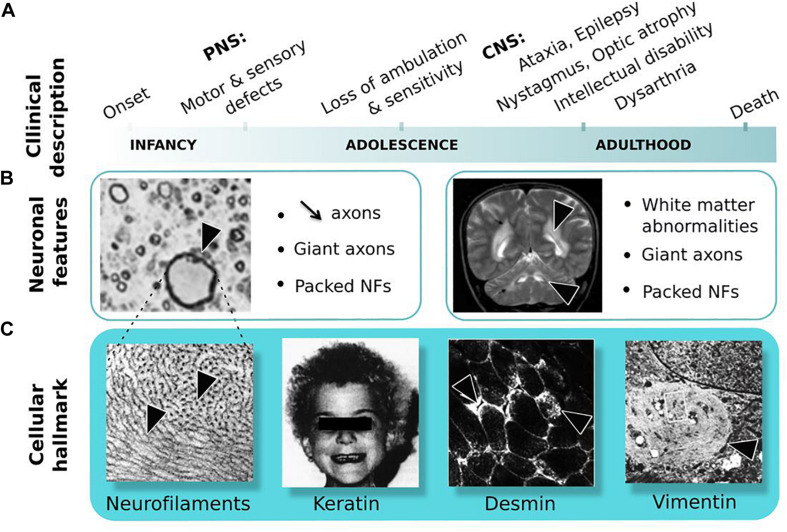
Giant Axonal Neuropathy. (A) Symptoms are shown in order of occurrence: from onset in childhood in the peripheral nervous system (PNS) to adulthood in the central nervous system (CNS). (B) Neuronal features: (left) nerve cross-section in the PNS shows reduced axonal content and giant axon; (right) magnetic resonance imaging of the brain reveals white matter abnormalities. Giant axons of CNS are not represented here. (C) Cellular hallmark: (from left to right) accumulation and altered distribution of neurofilaments (NFs) in giant axons, curled hair, desmin bundles in muscles and aggregates of vimentin in skin-derived fibroblasts. Copyright with permission from BioMed Central, Springer and Elsevier.
